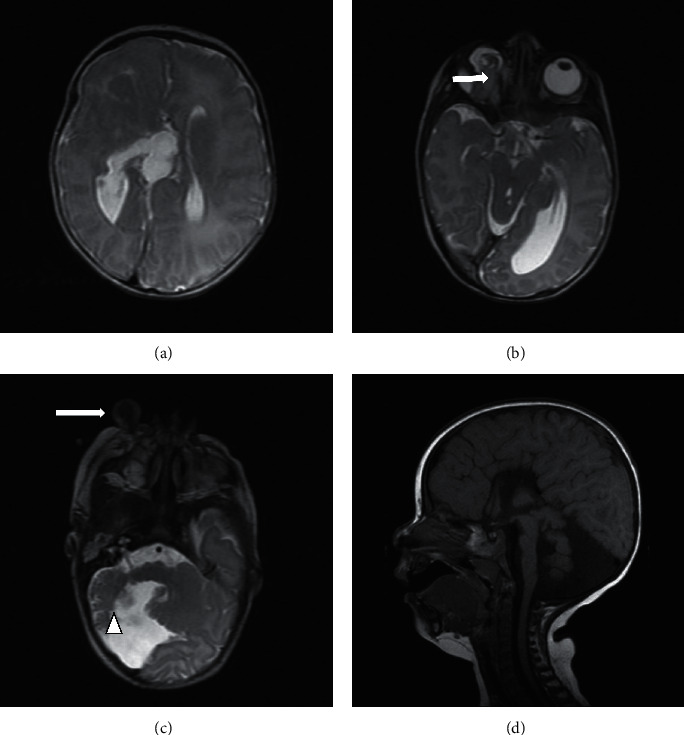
Axial images of T2W MRI demonstrated (a) colpocephaly and agenesis of the corpus callosum, (b) a mixed cystic solid structure in the right orbital cavity (black arrow), and (c) a cutaneous appendage in the right infraorbital area (white arrow), aplasia of the vermis, hypoplasia of the right cerebellar hemisphere (head arrow), and widening of the CSF space in the posterior fossa. Sagittal image of T1W MRI in midline showed (d) agenesis of the corpus callosum and cerebellar hypoplasia.
